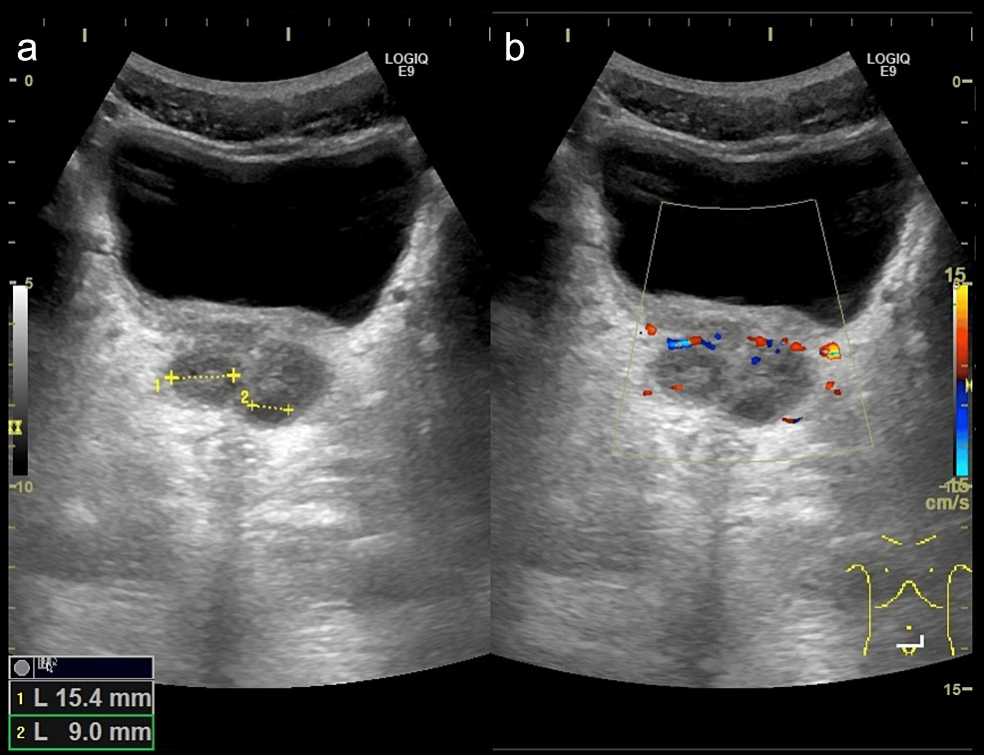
Ultrasonography images Ultrasonography revealed an enlarged prostate and multiple small cysts, which were 15.4 mm and 9 mm in diameter (a), and increased color flow (b).
